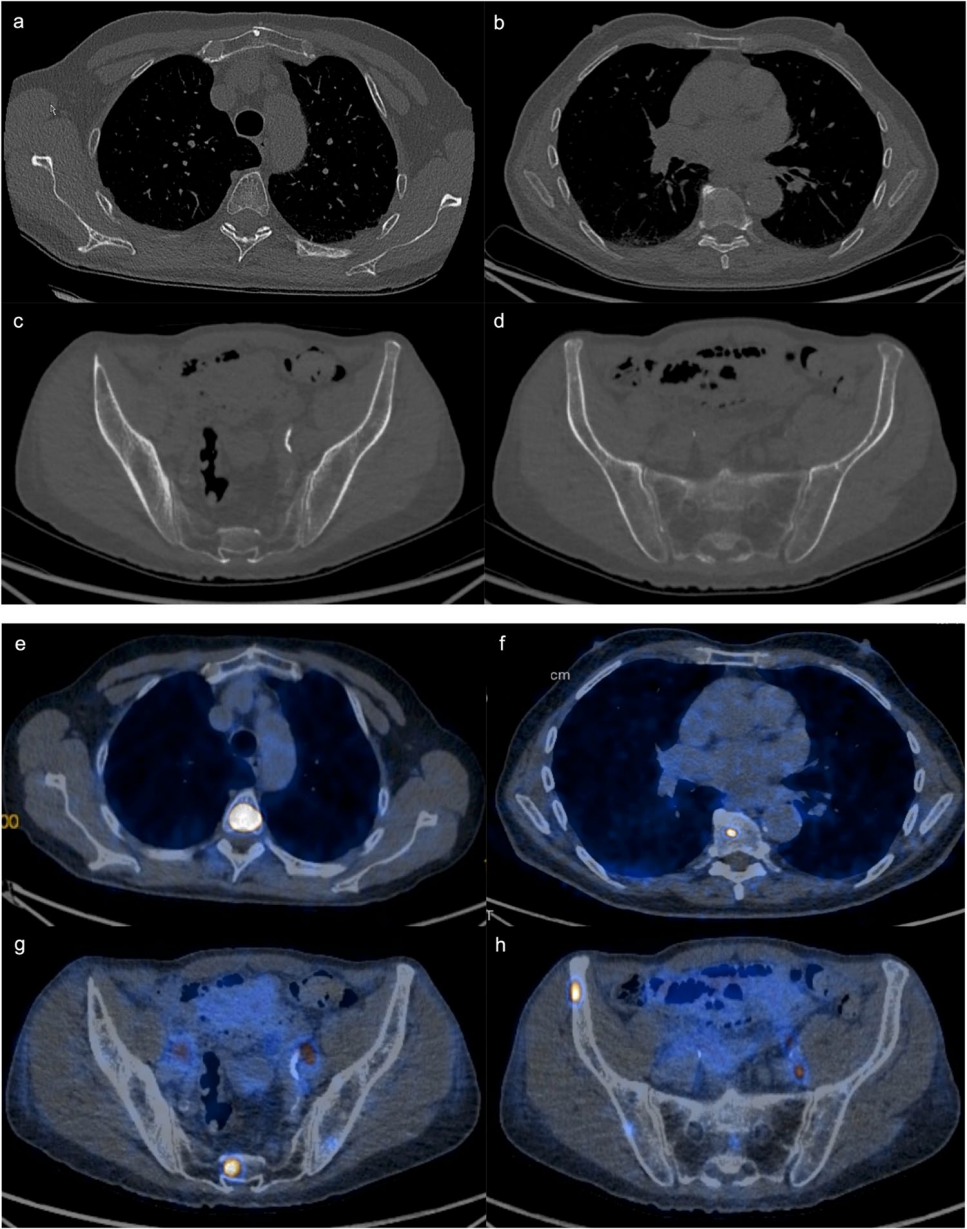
CT and corresponding PET/CT in three representative patients with metastatic bone disease from PCa. Can you identify the bone metastases in the upper (a) and mid (b) thoracic spine, the inferior part of the sacrum (c), and the right iliac bone (d) on CT only, without the additional metabolic information from PET? Corresponding PET/CT images clearly show high ^68^ Ga-PSMA uptake of the bone metastases in the aforementioned skeletal regions (e–h). Note additional ^68^ Ga-PSMA-positive lymph node metastases along the left iliac vessel axis (g, h)
